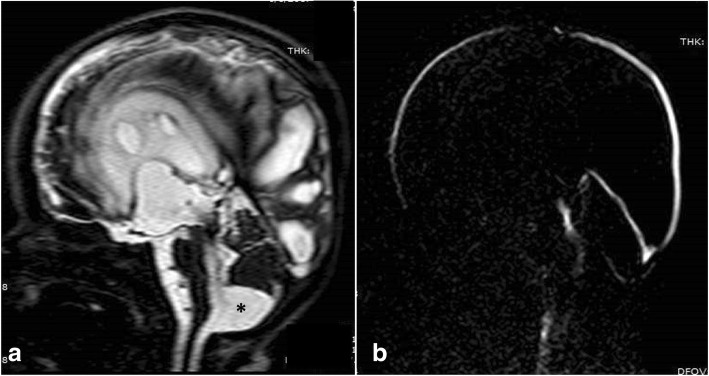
A 12-month-old girl with Blake’s pouch cyst. a 3D-DRIVE showing infracerebellar cyst (asterisk) associated with dilated third and fourth ventricles. b Phase-contrast MRI reveals absence of communication between the cyst and the posterior cervical subarachnoid space
